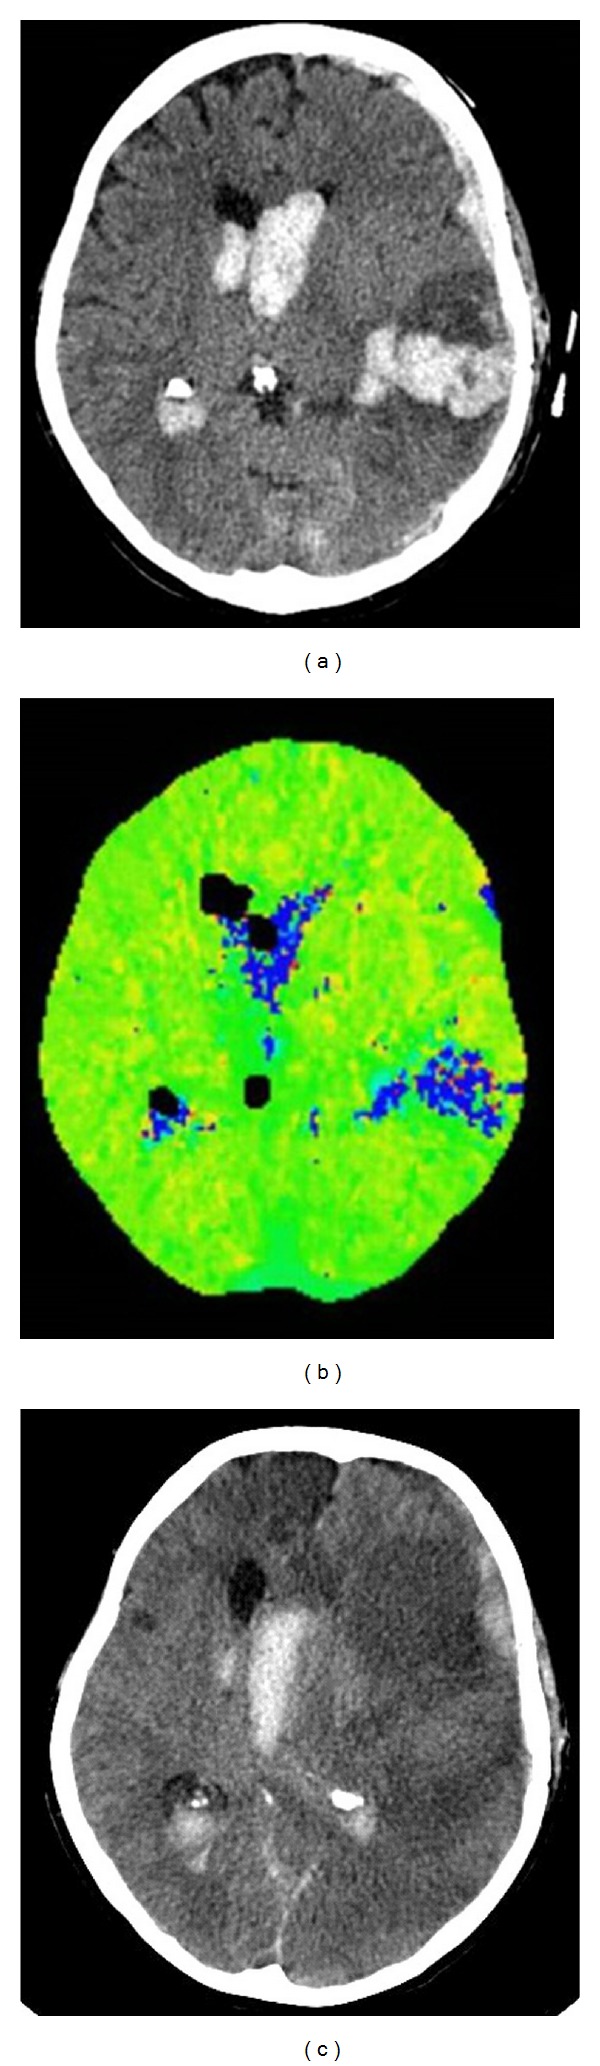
(a) and (b) native CT and perfusion-weighted CT scan at time of left-sided desaturation on day 5 without new ischemic stroke; (c) native CT scan four days later showing distinct left hemispheric ischemic stroke with midline shift.
